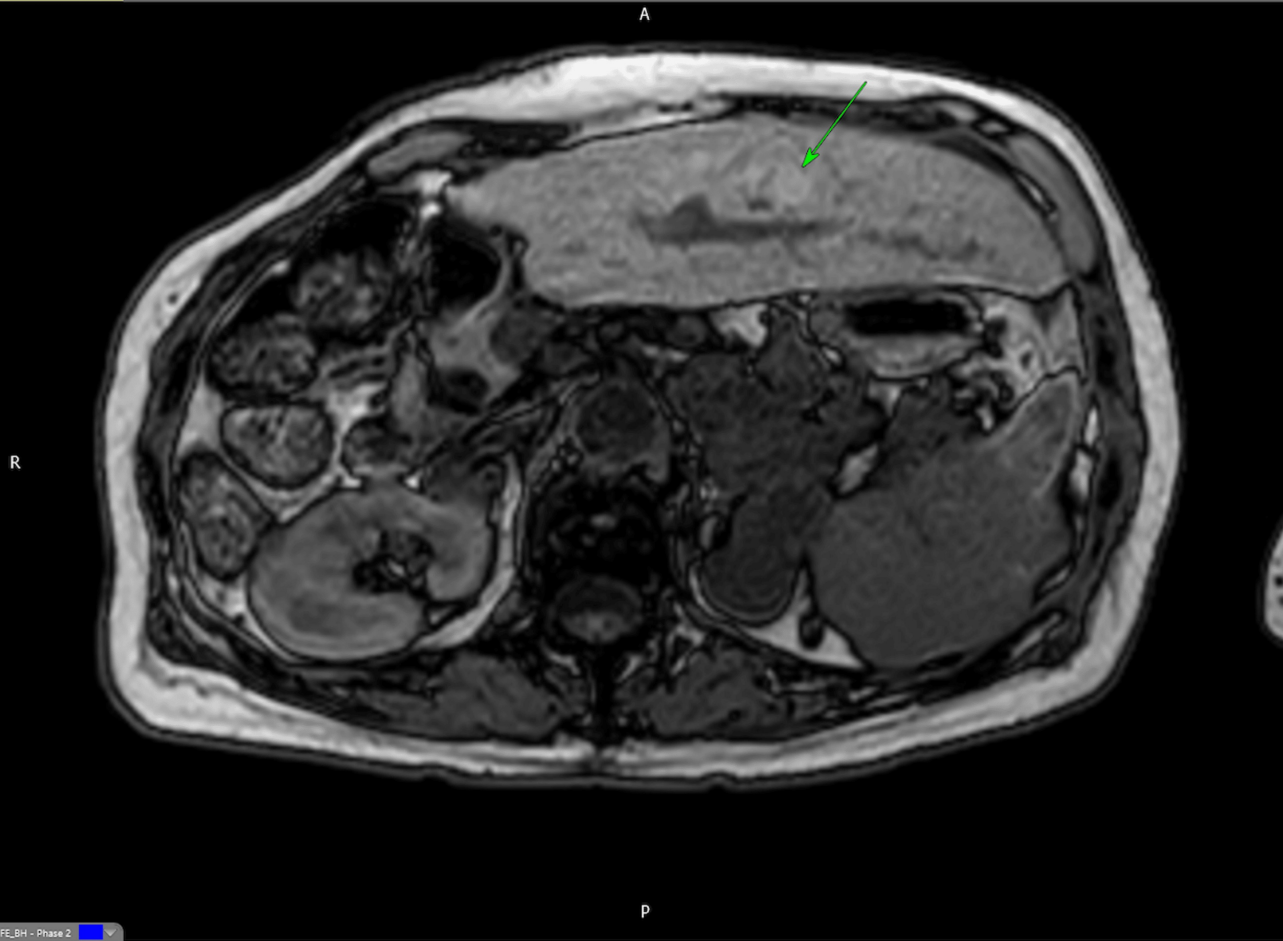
T1-weighted MRI of the liver The arrow indicates the area with the intrinsic high T1 signal
